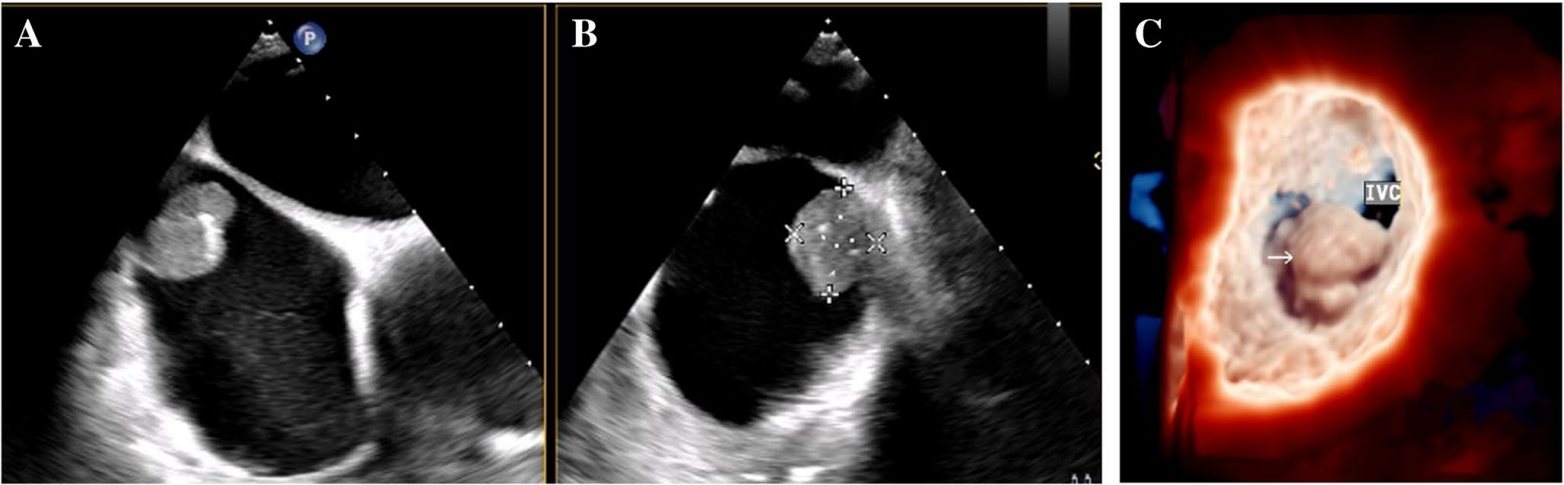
Transthoracic echocardiography (A and B) and transesophageal echocardiography 3D imaging (C) of right atrial myxoma
